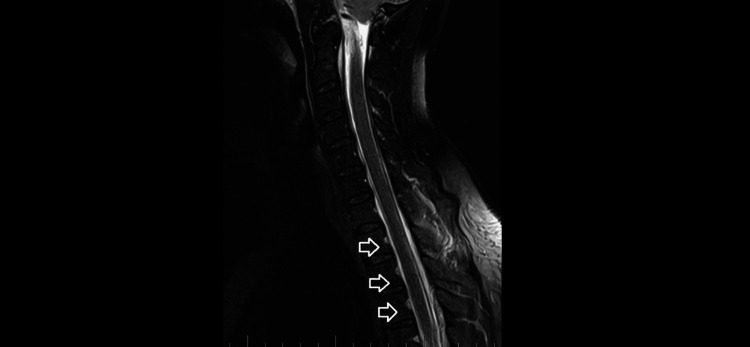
C spine MRI on March 5, 2023. Sagittal T2-weighted images. The white arrows show the regression of spinal longitudinal extradural fluid collection.
